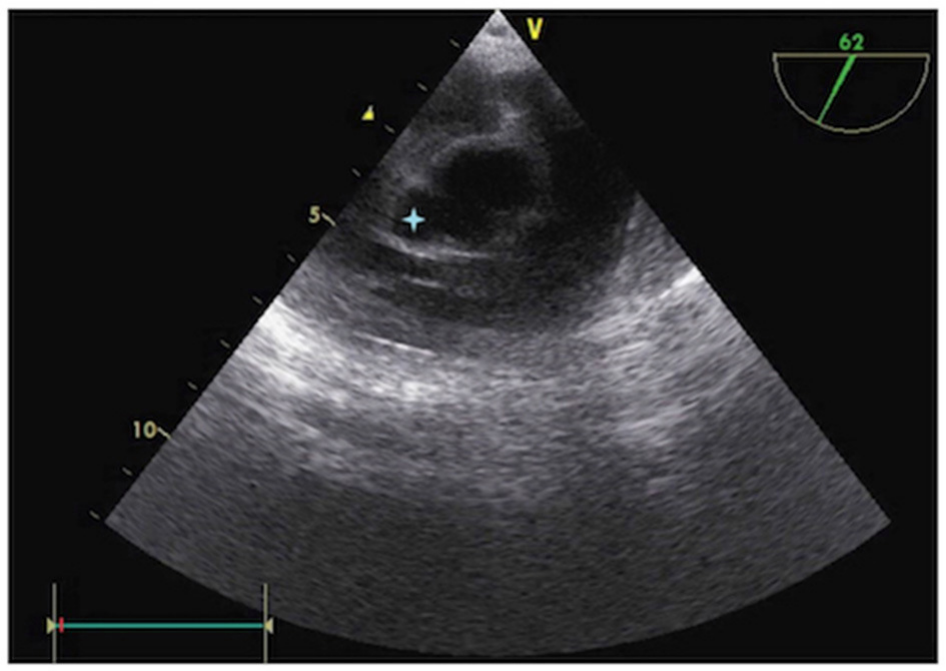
Preoperative transesophageal echocardiography (TEE) showing a para-aortic abscess (
